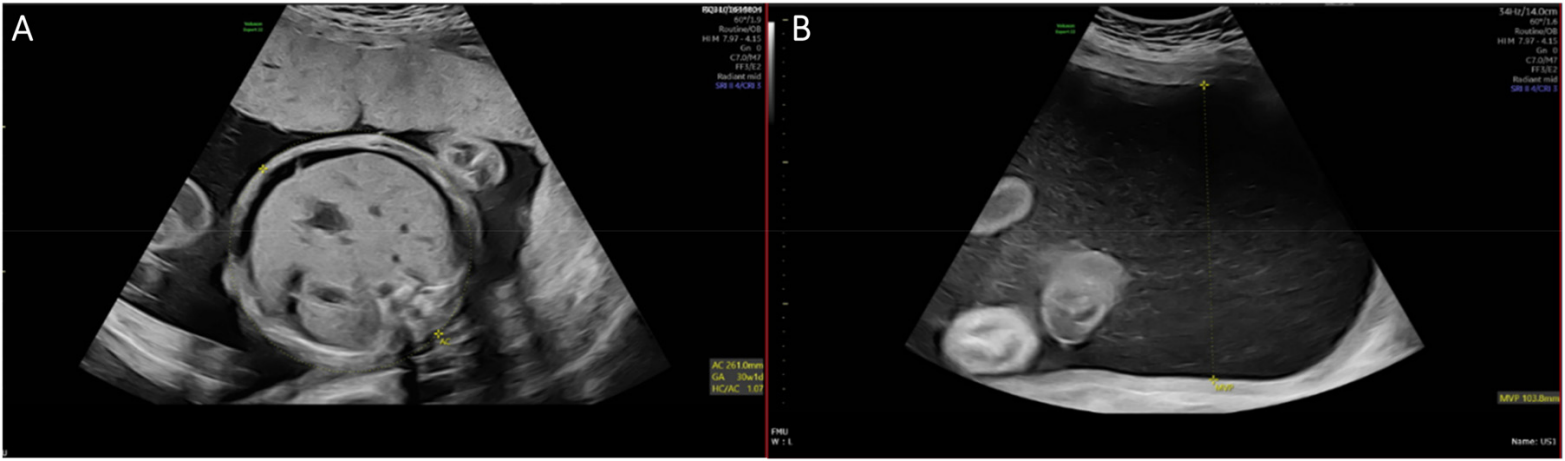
(A) Fetal ascites visualised on ultrasound. (B) Maximal vertical pool depth (MVP) measuring 10.4 cm indicating polyhydramnios.
